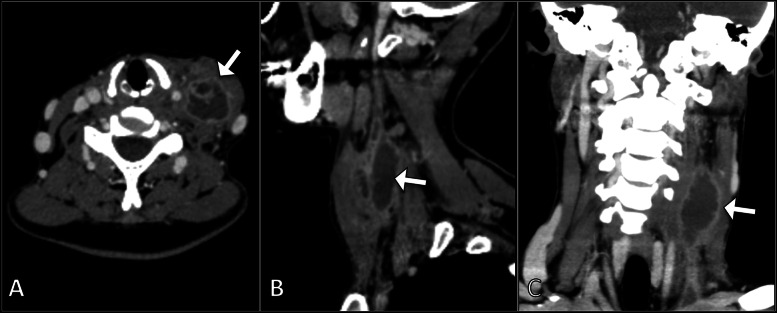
CT images of the neck in axial (A), sagittal (B), and coronal (C) planes demonstrate a distended caliber of the left internal jugular vein with an enhancing wall and intraluminal filling defect consistent with thrombophlebitis (arrows)
